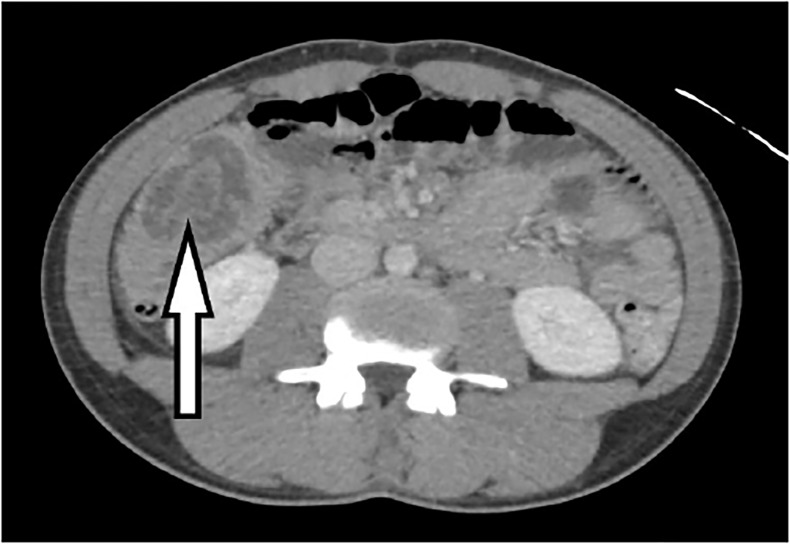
Example of the “target” sign (arrow) of intussusception as seen on the patient’s computed tomography.
